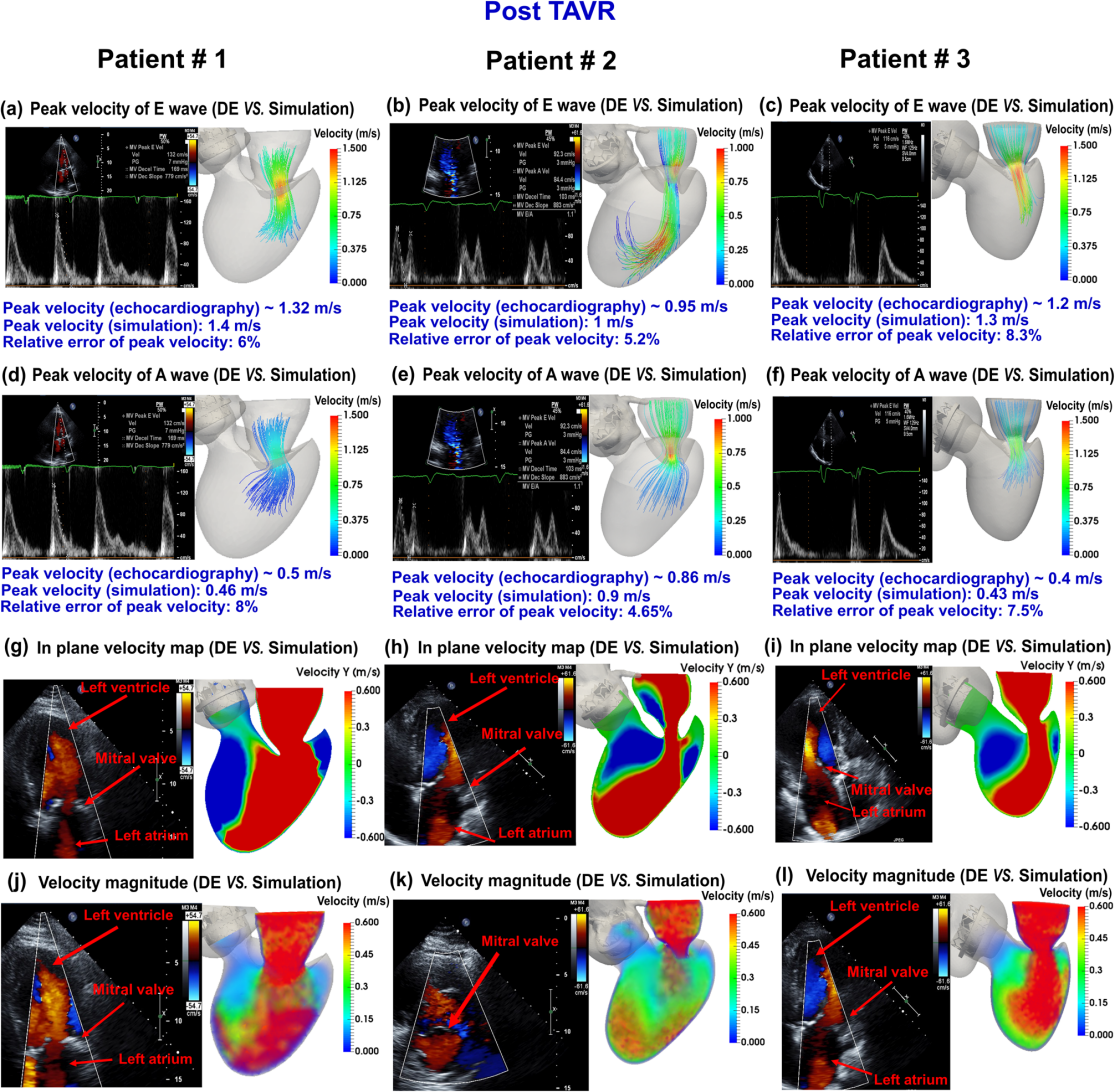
Validation*: Doppler-based LPM and FSI framework versus clinical Doppler echocardiography data in post-TAVR condition. (a–f) Trans-mitral velocity during diastole in patients #1 to #3; (g–i) Left ventricle flow (apical view) during diastole in patients #1 to #3; (j, l) Trans-mitral and left ventricle flow (apical view) during diastole in patients #1 and #3; (k) Mitral valve flow (parasternal short axis view) in patient #2.
